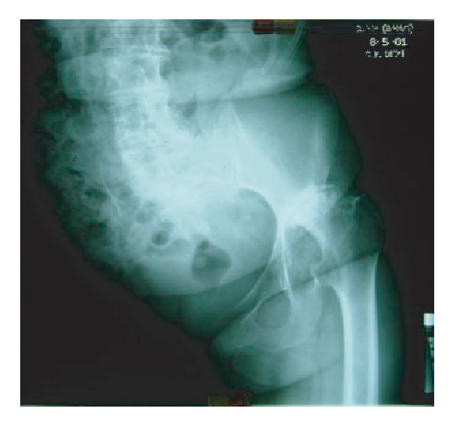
AP X-ray of the pelvis showing the result 9 years following
